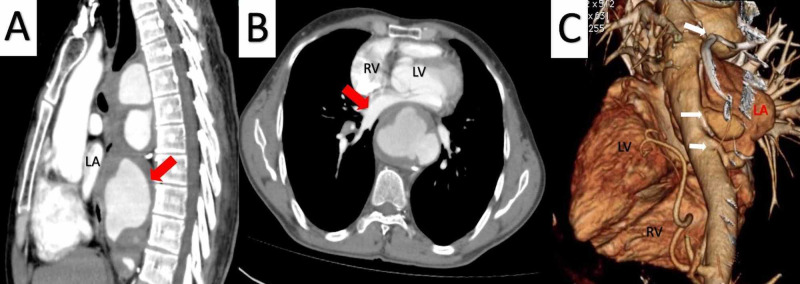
(A) Contrast enhanced sagittal computed tomographic imaging of thorax showing multiple, complex, multilobulated aneurysms arising from arch, continuing to descending thoracic aorta. Lowermost large aneurysm of size 4.9 x 5.2 x 3.4 cm is seen compressing left atrium (LA). Mural thrombosis is noted (Red arrow). (B) Contrast enhanced axial computed tomographic imaging of thorax (D8-D9 vertebra) showing multilobulated wide aneurysm of size 4.9 x 5.2 x 3.4 cm with mural thrombus compressing the left atrium (LA) anteriorly. Red arrow points to left atrium (LA). LV is left ventricle and RV is right ventricle. (C) Volumetric rendered imaging of thorax revealing multiple saccular aneurysms arising from descending aorta (white arrows) in relation to left atrium (LA).
